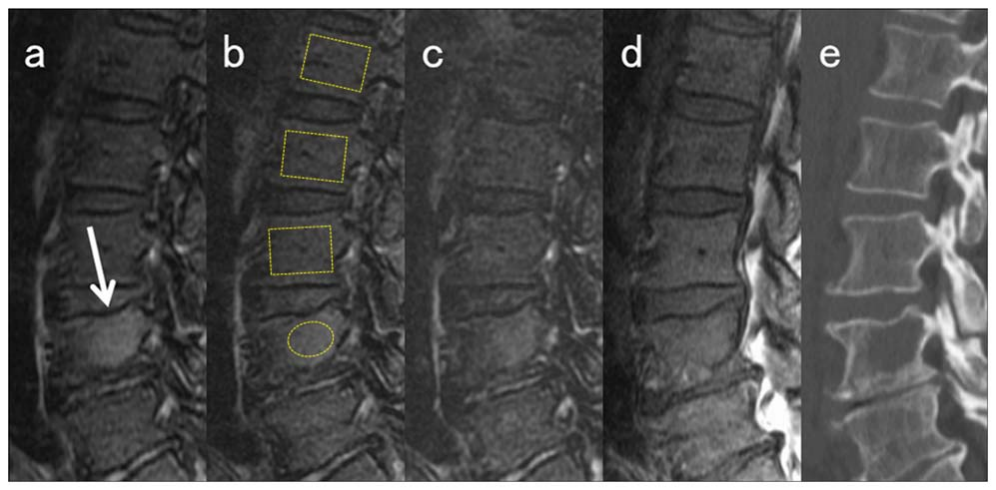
Sagittal MRI in a 65-year-old man with combined focal and diffuse infiltration pattern (BMPC%, 71%). a) Water image of IDEAL; b) FS-T_2_ FSE; c) STIR; d) T_1_ FSE; and e) CT. ROIs of lesion and background BM used for the percent contrast computation are demarcated in the images. The actual ROIs of BM are placed in similar locations in the midsagittal images. The focal myeloma lesion in L4 is seen as a slightly high intensity signal on fat-suppressed images (white arrows) but is less conspicuous compared to the lesion in Figure 3. Among the three fat-suppressed images, signal hyperintensity of the lesion is less conspicuous in STIR (c) than in water image of IDEAL (a) or FS- T_2_ FSE (b). The focal lesion is not detected on T_1_ FSE (d).
